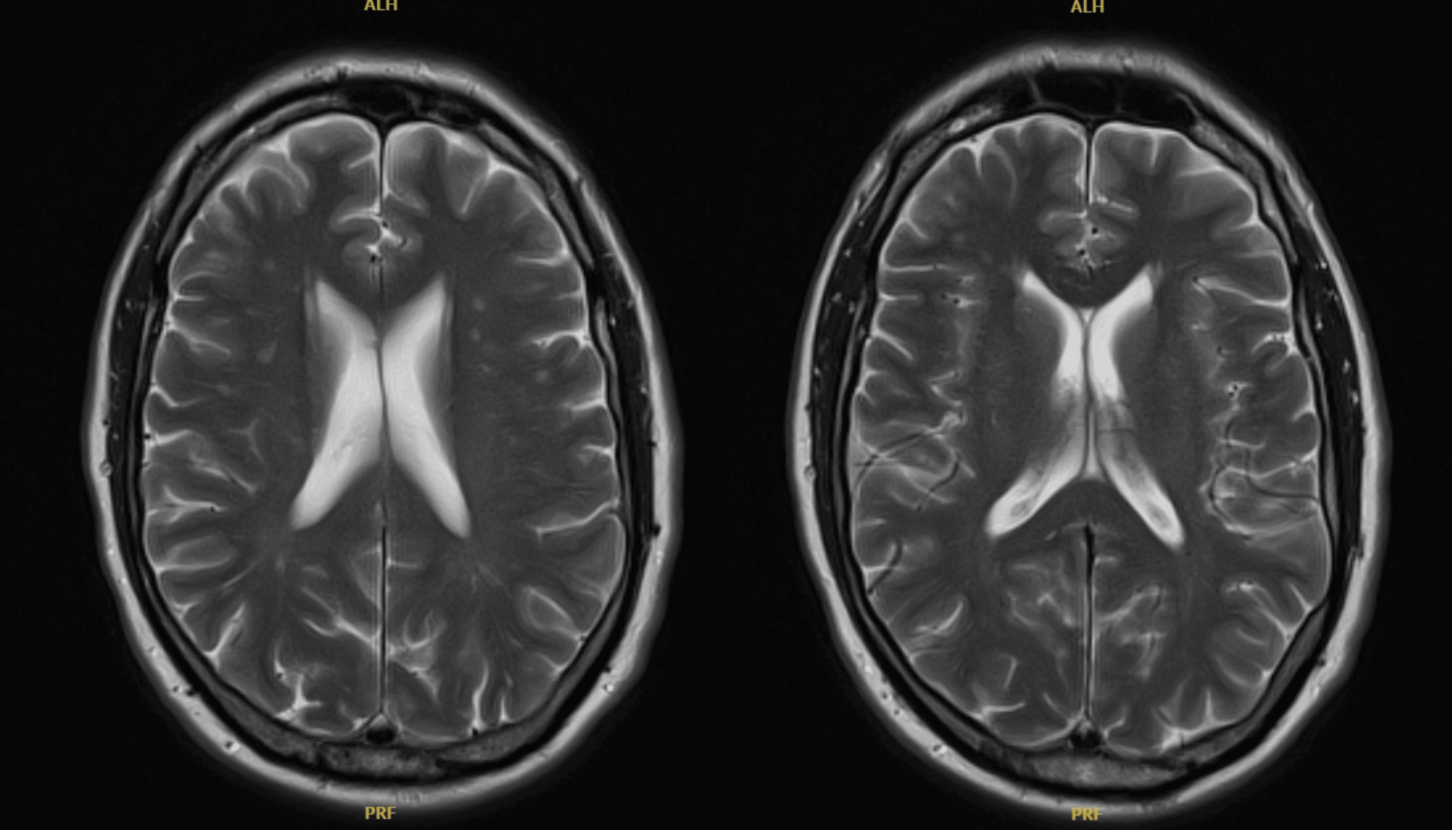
Axial brain MRI showing normal brain tissue and well-preserved ventricular structures. MRI: magnetic resonance imaging
